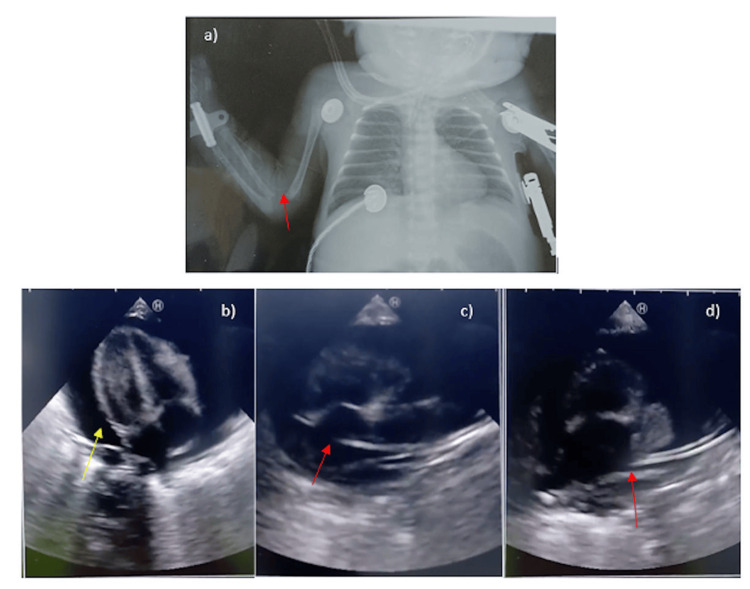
Echocardiogram and chest radiograph of Case 1 a) Supine chest radiograph following PICC insertion, the catheter tip was not visualized; b) PCE; c) PICC tip positioned within the right atrium; d) PICC tip located at the junction of the superior vena cava and the right atrium after the catheter was withdrawn. PICC: Peripherally inserted central catheter; PCE: Pericardial effusion
